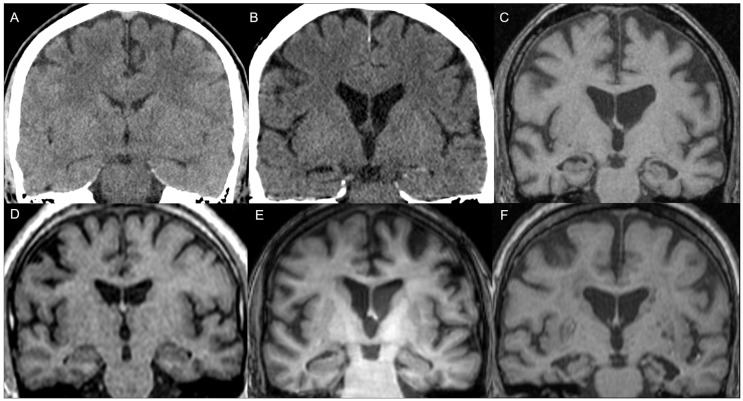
Examples of Medial Temporal Lobe Atrophy (MTA) scores in the sample. Bilateral MTA 0 in a 28-year-old patient who presented with auditive hallucinations on CT (A) and MRI (D); right MTA 1 and left MTA 0 on CT in a 40 year-old patient who presented with auditive and visual hallucinations (B) and in a 48-year-old patient who presented with auditive hallucinations on MRI (E); left MTA 2 and right MTA 1 on MRI in a 65-year-old patient who presented with auditive and visual hallucinations (C); right MTA 2 and left MTA 3 on MRI in a 77-year-old patient who presented with auditive hallucinations (F).
